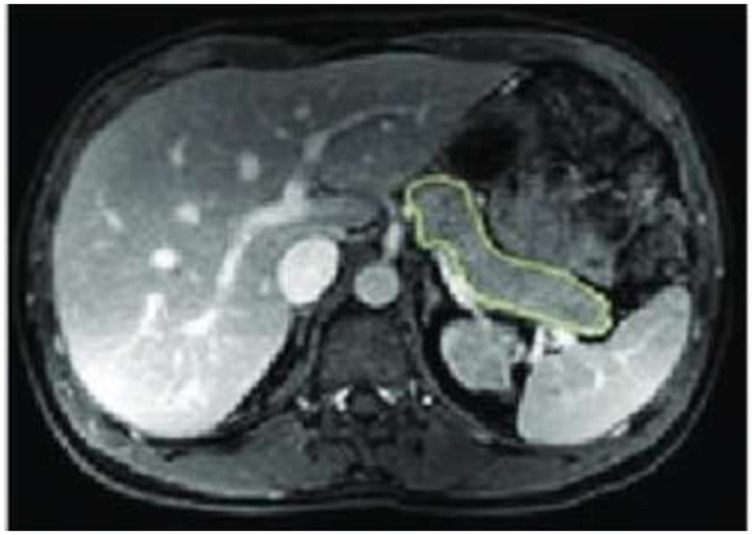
Abdominal MRI showing pancreatic size drawn by hand in the transaxial plane.
